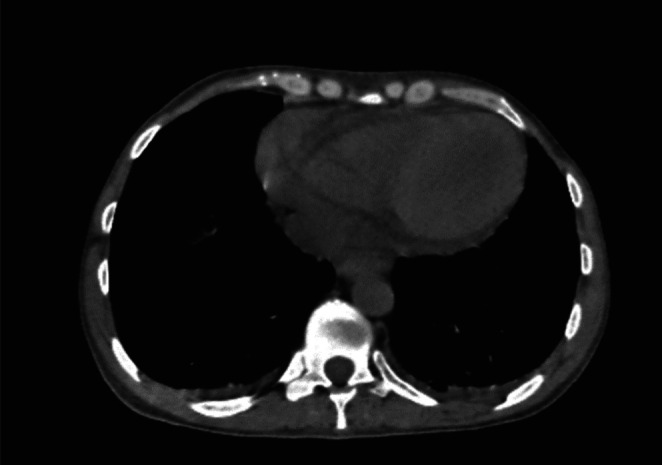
Chest CT scan showing mild bilateral pleural effusion and pericardial effusion (8 mm).
